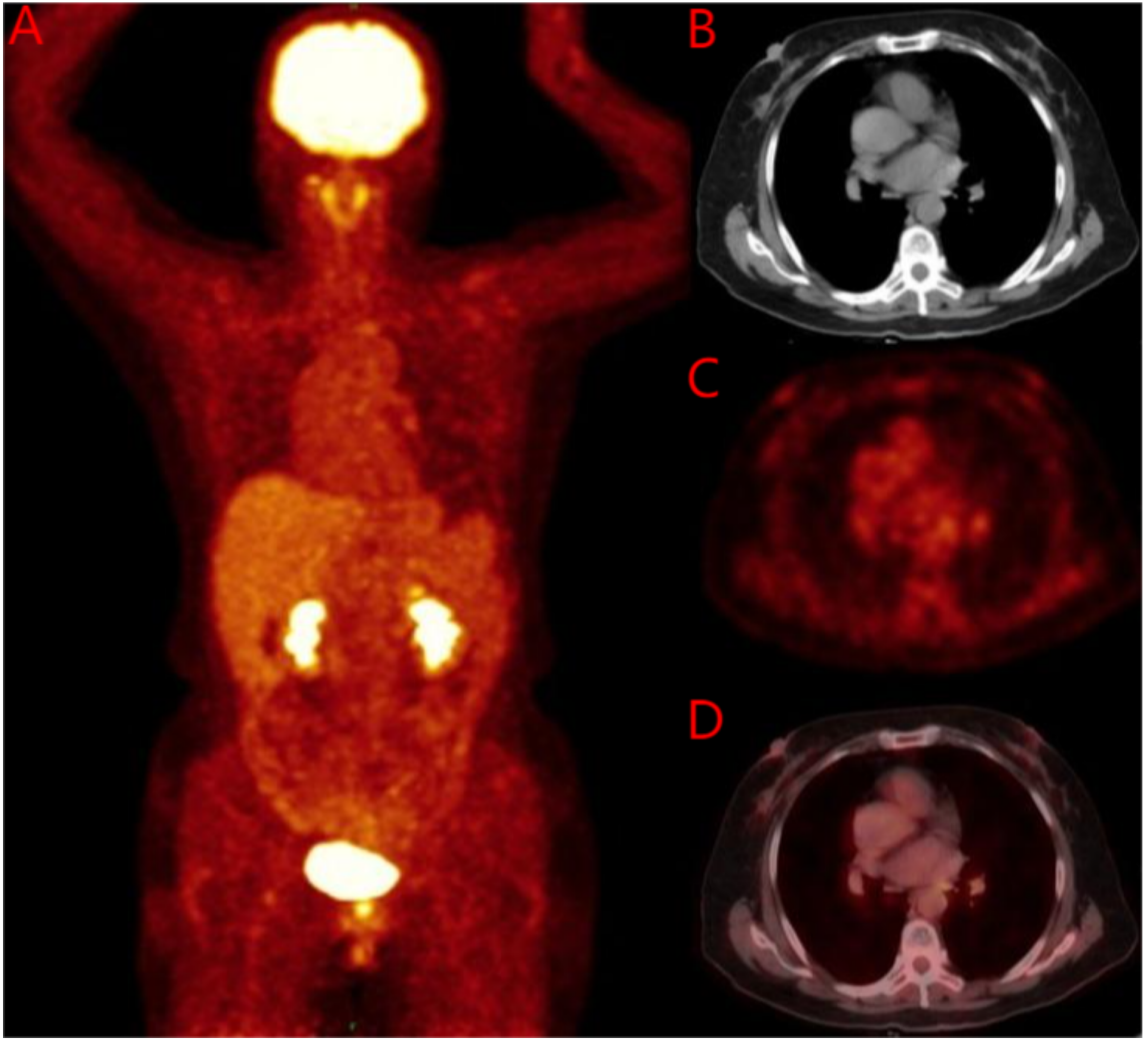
18F-FDG PET/CT before breast treatment. (A) Whole body image of 18F-FDG PET/CT; (B) No soft tissue mass was found on breast CT; (C) No soft tissue mass was found on breast PET; (D) No soft tissue mass was found on breast MIP. 18F-FDG PET/CT, 18F-flurodeoxyglucose positron emission tomography-computed tomography; MIP, Maximum Intensity Projection.
